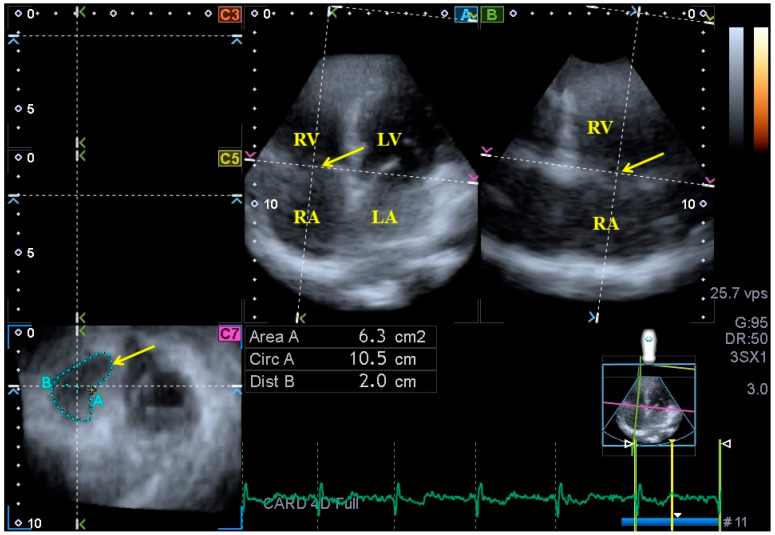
Assessment of the tricuspid annulus extracted from a three-dimensional full-volume dataset is presented: apical four-chamber view (A); apical two-chamber view (B); and a cross-sectional view at the level of the tricuspid annulus optimized in apical four- and two-chamber views (C7). The yellow arrow represents the tricuspid annular plane. Abbreviations: LA = left atrium, LV = left ventricle, RA = right atrium, RV = right ventricle, Area = TA area, Circ = TA perimeter, Dist = TA diameter.
